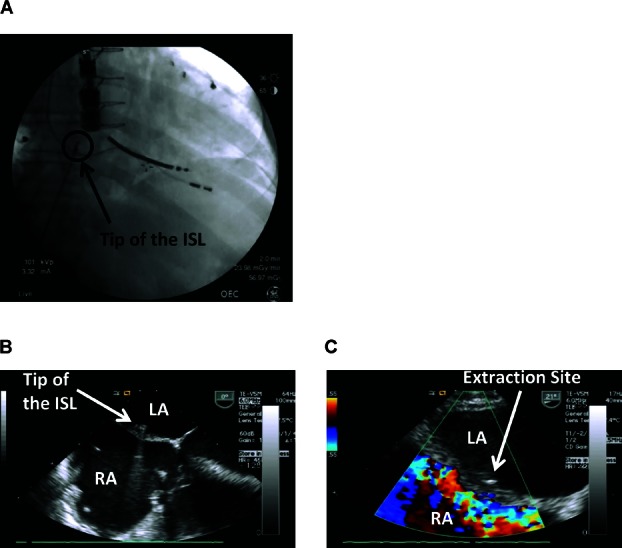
(A) Fluoroscopy image showing manual traction being applied to ISL with a locking stylet via the femoral vein using the inferior approach. (B) TEE image showing reverse tenting while traction is being applied to the ISL. (C) TEE image showing the absence of a residual atrial septal defect immediately following ISL extraction. ISL = implantable sensor lead; LA = left atrium; RA = right atrium; TEE = transesophageal echocardiography.
